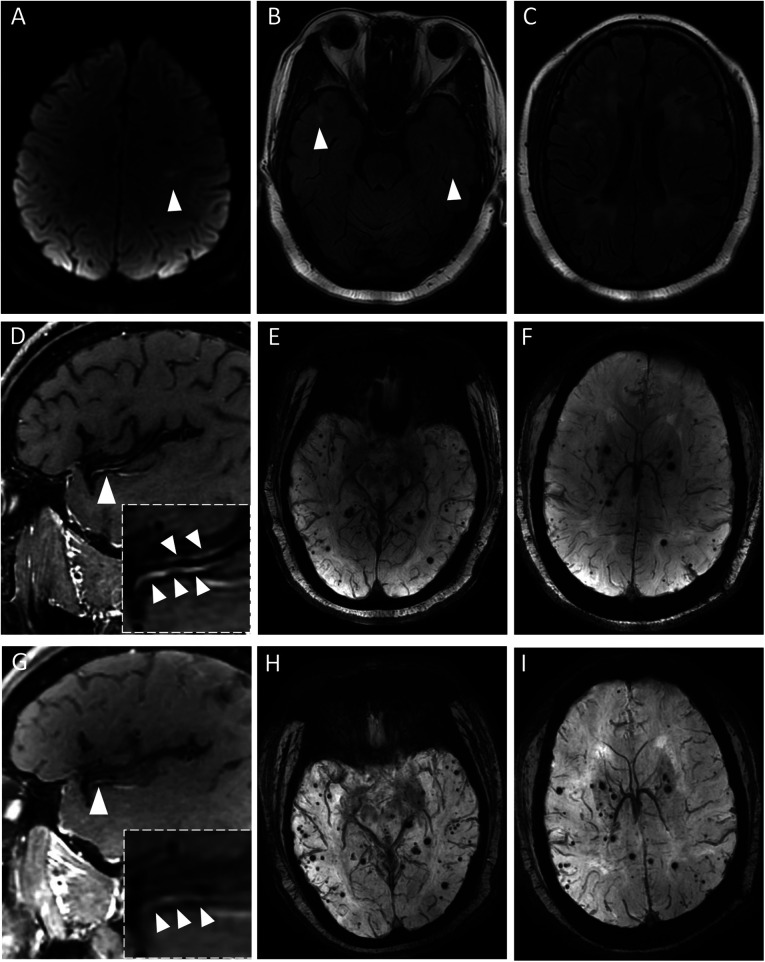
Neuroimaging features at admission and serial high-resolution vessel wall MRI (VWMRI) findings. (A) DWI hyperintensity in the left centrum semiovale showed acute ischemic stroke at admission. (B,C) white matter hyperintensities (WMH) were observed in bilateral temporal lobes, subcortical and periventricular regions on T2-FLAIR. (D) VWMRI revealed homogeneous, concentric vascular wall enhancement with thickening along a branch of the right MCA. (E,F) Cerebral microbleeds (CMBs) in the frontal lobe, parietal lobe, temporal lobe, occipital lobe at admission on susceptibility-weighted imaging (SWI). (G) Follow-up VWMRI at 14 months demonstrated persistent enhancement in the same branch of the right MCA. (H,I) Number of CMBs increased in the follow-up SWI.
